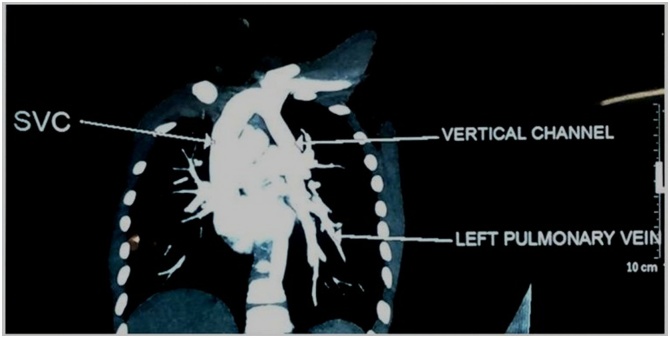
CT Pulmonary Angiography (CTPA) showing the left pulmonary veins joining to form the vertical vein before draining into the superior vena cava (SVC).
